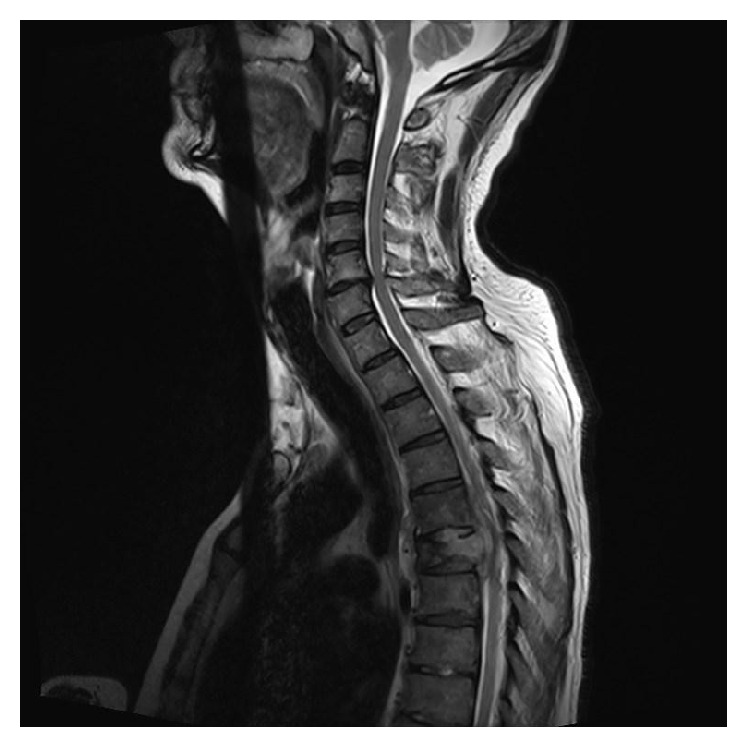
MRI scan, sagittal T2 weighted image, showing posterior soft tissue mass at the level of T5 with associated pathological fracture and diffuse paravertebral oedema. There is evidence of moderate compression of the spinal cord.
